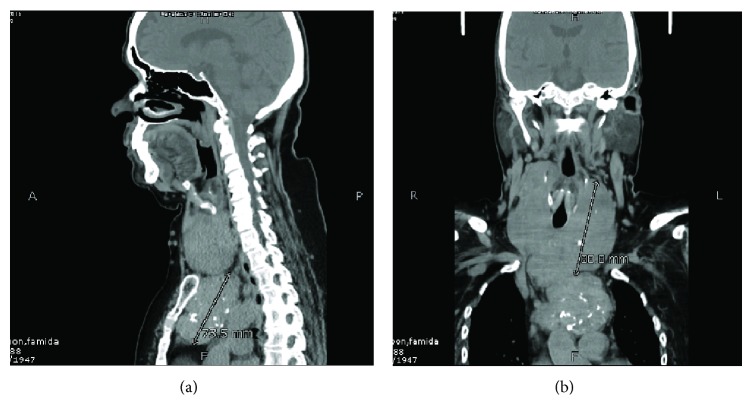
CT scan demonstrating retrosternal extension of thyroid mass in a sagittal and a coronal section.
